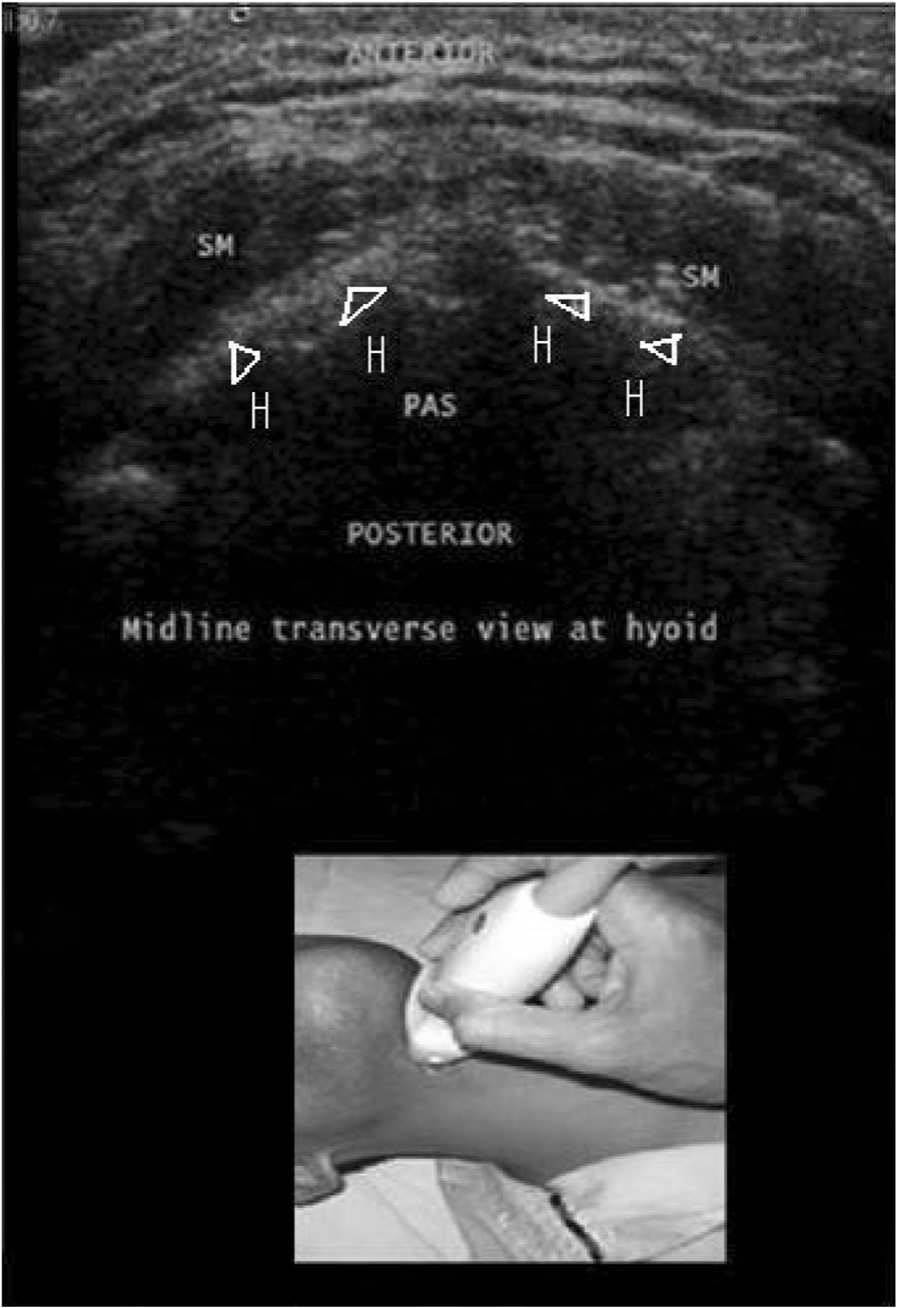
Sonogram of the hyoid bone (H) on a midline transverse view using a linear transducer. The hyoid bone is shown as an inverted, hyper-echoic U. PAS, posterior acoustic shadow; SM, strap muscles. Reprinted with permission from Wolters Kluwer [42]
